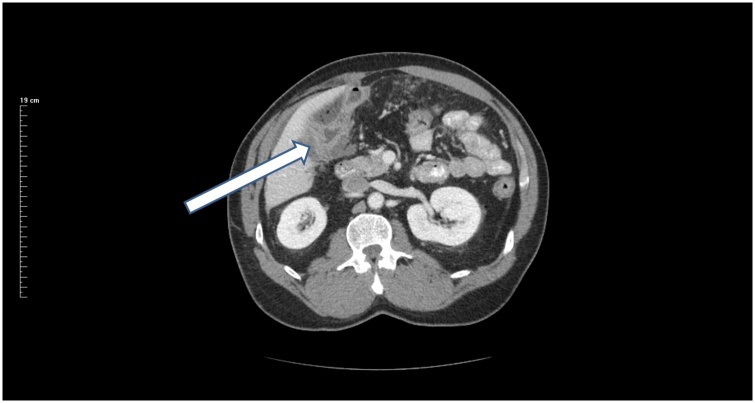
CT slice 4 months postoperatively through the upper abdomen. At the time of resection of recurrent cancer, tumor was located on the posterior surface of the liver. This anatomic site was a close margin of resection of the primary ascending colon cancer.
